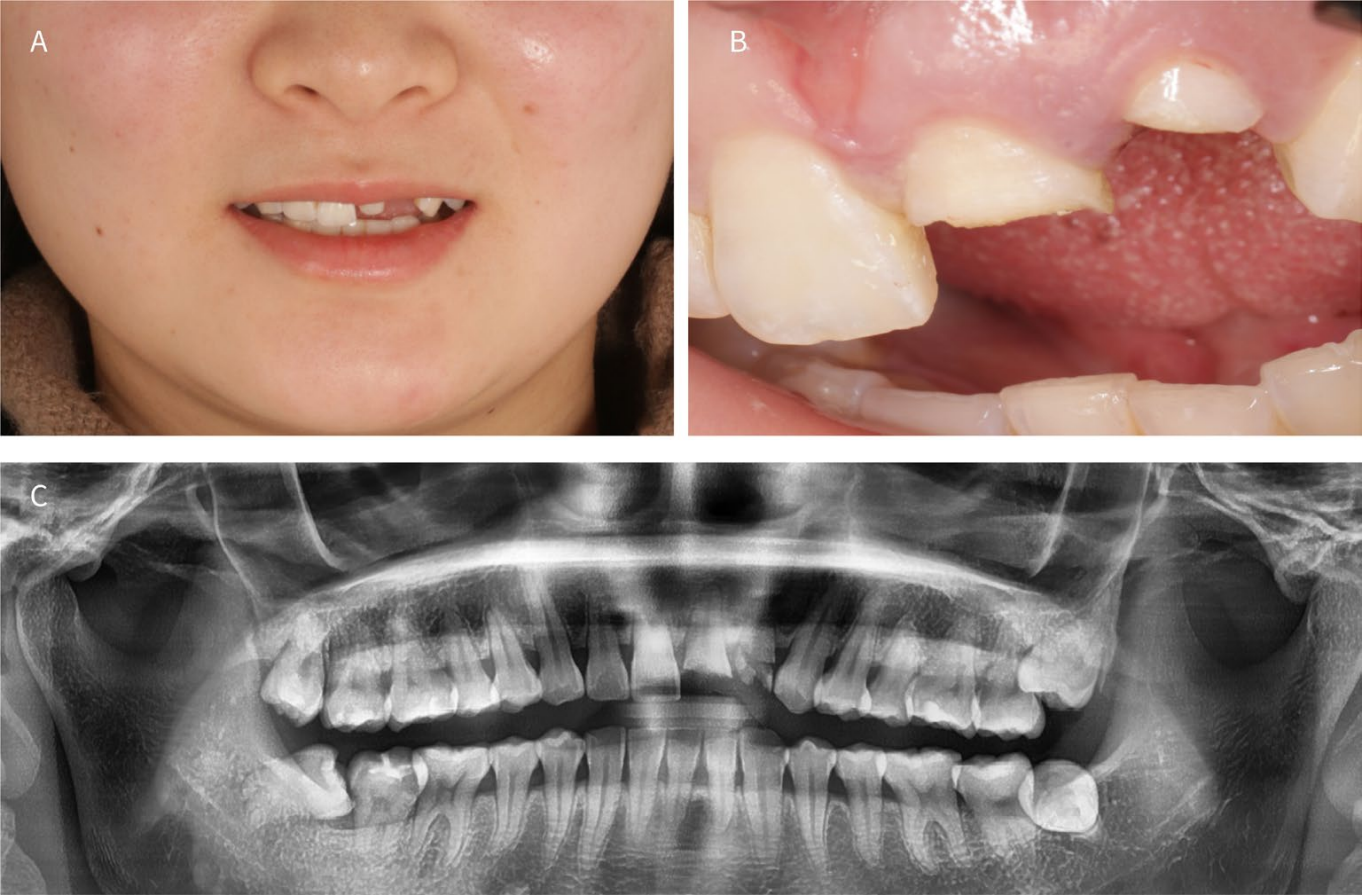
(A) Preoperative smile view; (B) Clinical intraoral examination before surgery; (C) Preoperative panoramic radiograph.
